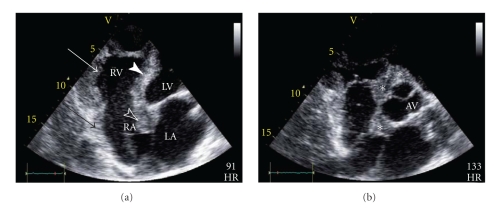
Apical two-dimensional four-chamber (a) echocardiogram showing marked thickening of the right ventricle (arrow), right atrium (empty arrow), interatrial septum (empty arrowhead), and basal to mid interventricular septum (arrowhead) associated with a pericardial effusion. Aortic valve short-axis view (b) demonstrating that the thickened tissue (asterisk) extends around the root of aorta. RA indicates right atrium; LA, left atrium; RV, right ventricle; LV, left ventricle; AV, aortic valve.
